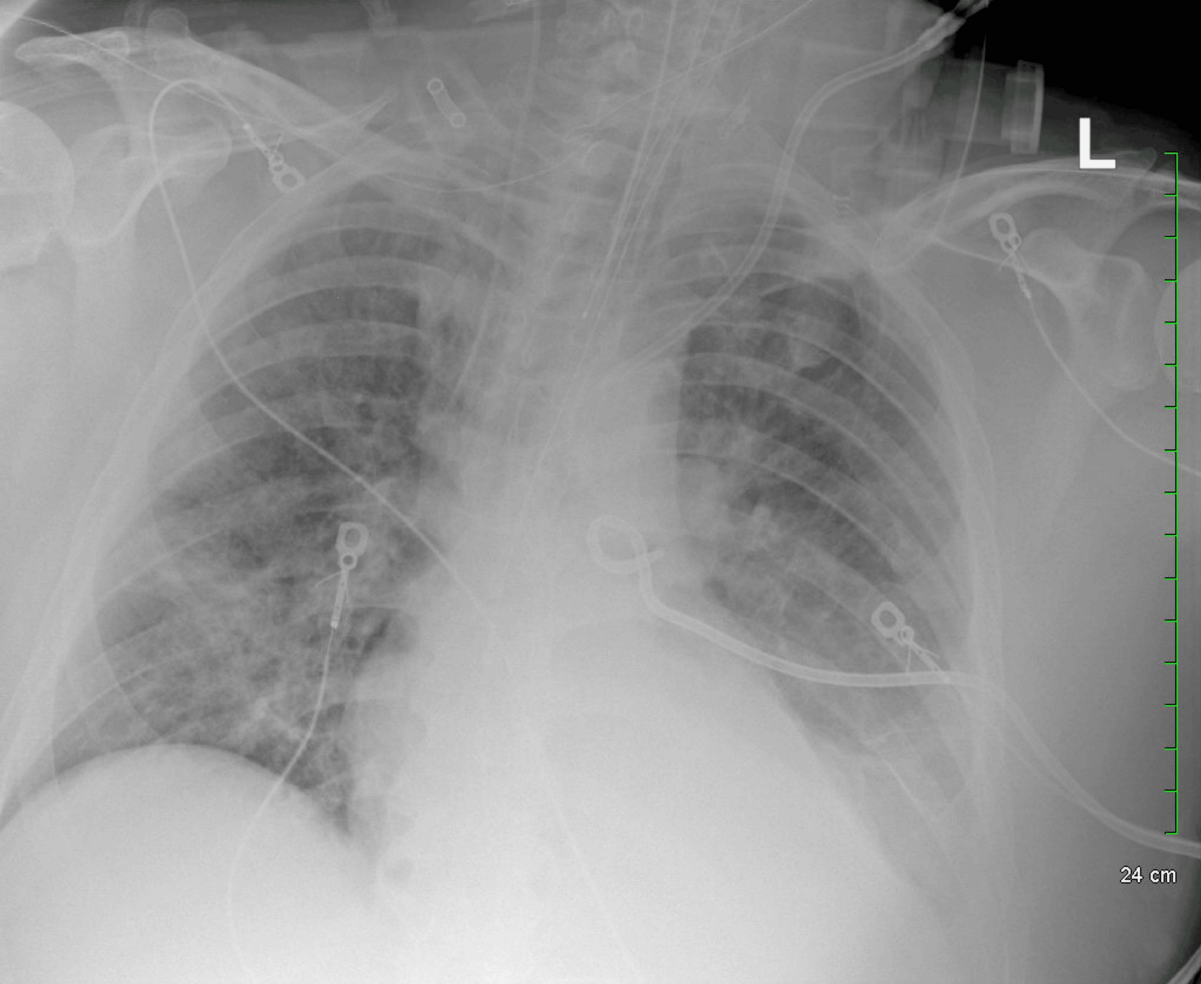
Day 4 chest X-ray, improving infiltrates and left-sided effusion
